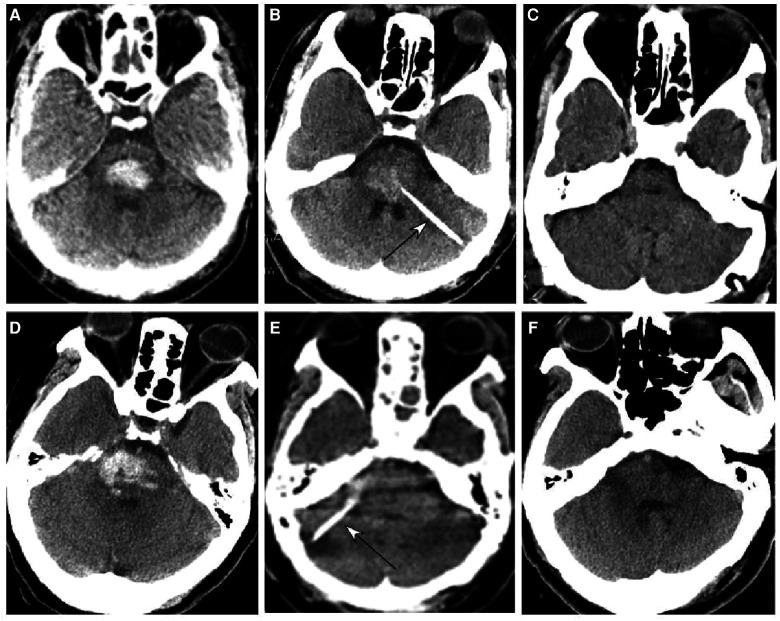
Brainstem hemorrhage treated with stereotactic aspiration. (A–C) A 59-year-old man had a sudden onset of unconsciousness 2 h before presentation. (A) Computed tomography (CT) revealed brainstem hemorrhage. Before the operation, he was on mechanical ventilation and in a moderate state of coma with a Glasgow Coma Scale (GCS) score of 5 (E1VTM4). (B) Stereotactic aspiration was performed (arrow). The arrow indicates the aspiration needle. On the third day following surgery, he regained consciousness and could get down from the bed with a GCS score of 14 (E4V4M6) and a Modified Rankin Scale score of 3. (C) Five months after surgery, the patient could walk and live without assistance. (D–F) A 65-year-old woman had a sudden loss of consciousness for 1 day. (D,E) CT imaging showed brainstem hemorrhage. Before surgery, she was in a moderate state of coma with GCS 6 (E2VTM4) and on mechanical ventilation (D,E). (F) Stereotactic aspiration was performed (arrow). After the surgery, the patient became conscious and could move her hands with a GCS score of 10 (E4VTM 6) and a modified Rankin Scale score of 4.
